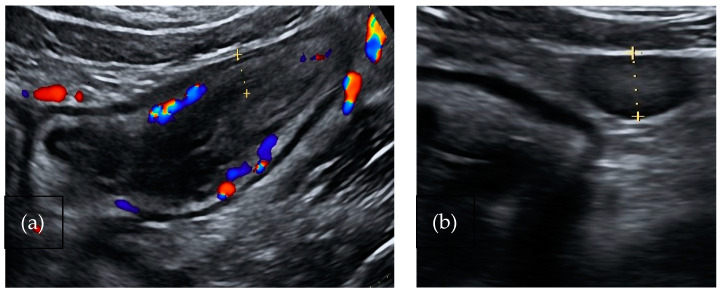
A 37-year-old-man affected by Campylobacter infection. (a). Bowel ultrasound shows thickening of the right colon wall (5.8 mm) with active inflammation (color flow Doppler signals in both the bowel wall and surrounding mesenteric fat). (b). Presence of lymphadenopathy.
